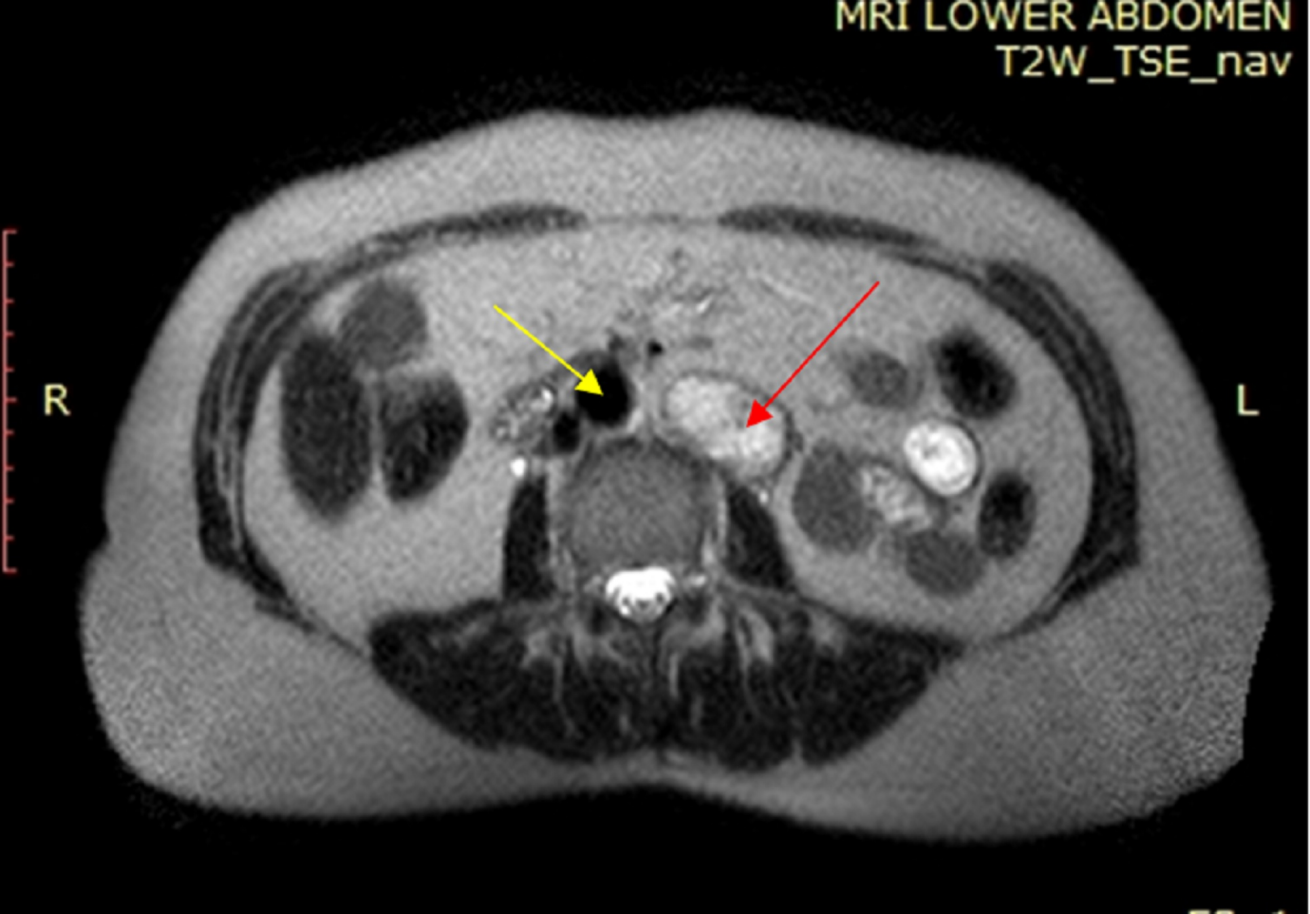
Preoperative T2-weighted axial MRI of the abdomen The image shows the lesion (red arrow) demonstrating a heterogeneous hyperintense signal, located on the left side of the aorta (yellow arrow) MRI: magnetic resonance imaging
